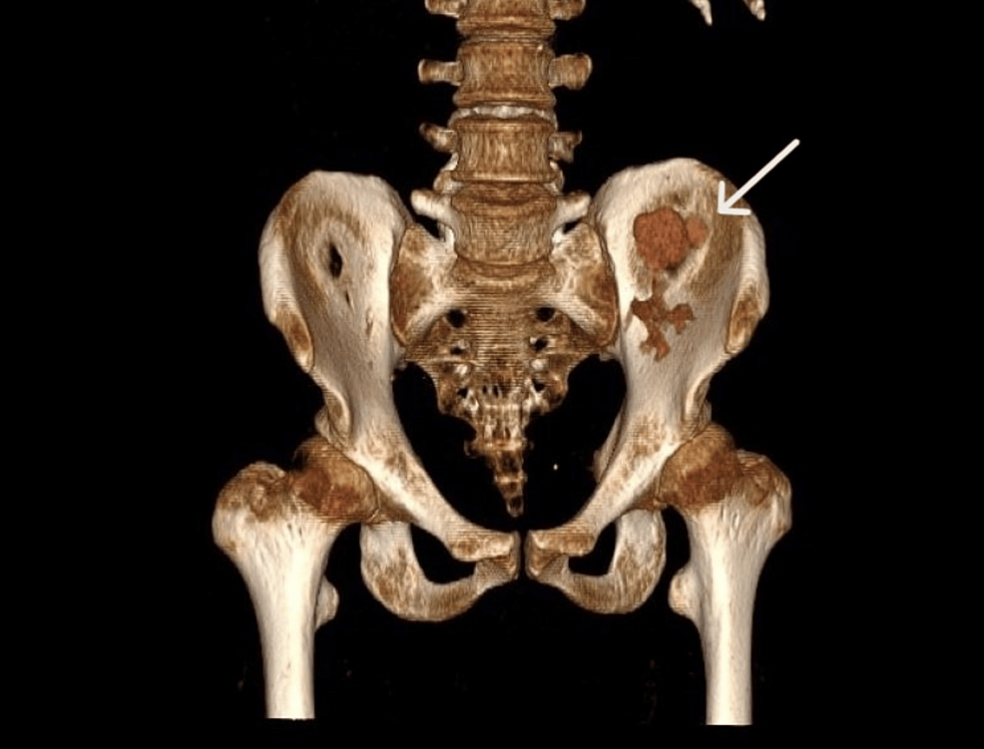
CT urography showing the dilatated upper calyx.
